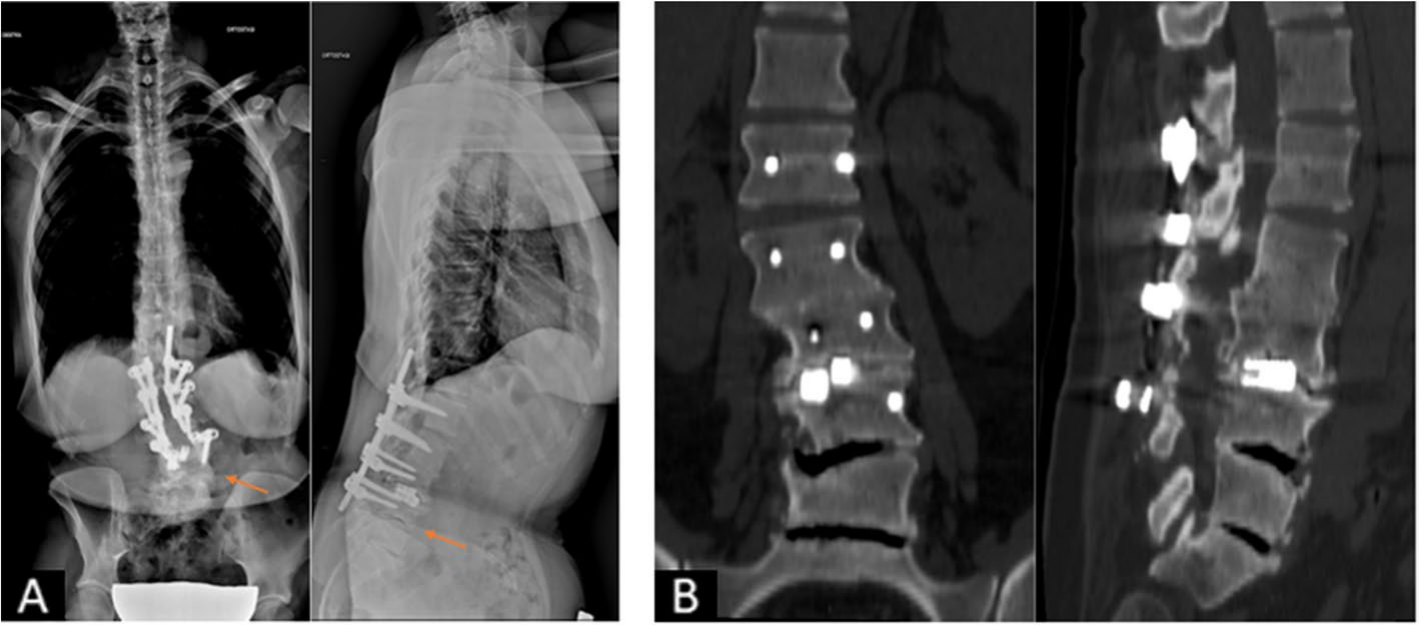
A and B: The x-rays (A) and CT scan (B) obtained in December 2012 showed a DJK an an erosion of the L5 endplate. Despite an anterior bony fusion from L2 to L4, the PLIF cage was clearly subsided, possibly due to the impaired bone structure in the endplates of L3 and L4
